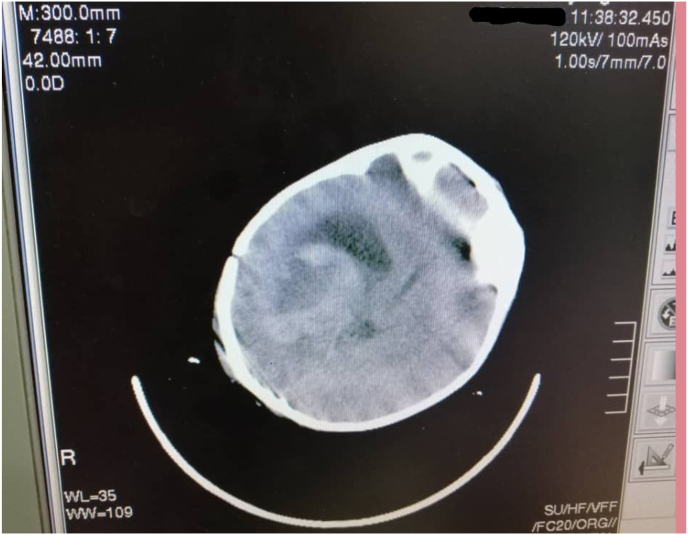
Computed Tomography showing a heterogeneous mass in the right lateral ventricle with marked midline shift.
